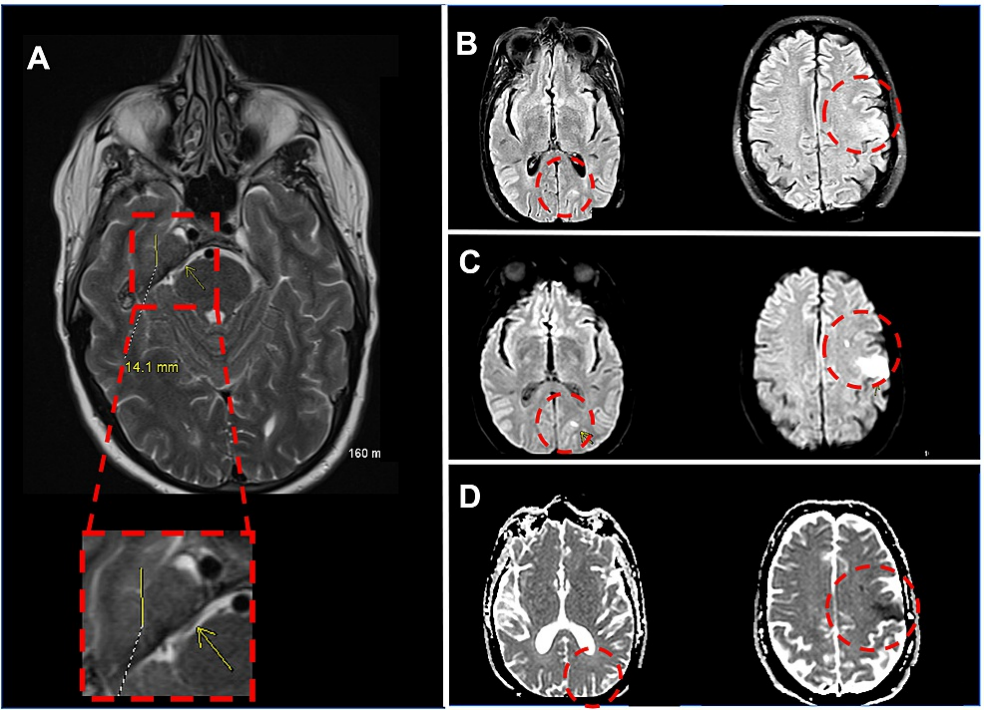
MRI of the brain A: 1.4 cm mass at the level of the posterior margin of Meckel's cave. B: Flair showing multiple, left-sided, subacute cardioembolic strokes. C: Diffusion-weighted imaging (DWI) showing multiple, left-sided, subacute cardioembolic strokes. D: Antibody-drug conjugate (ADC) showing multiple, left-sided, subacute cardioembolic strokes.
